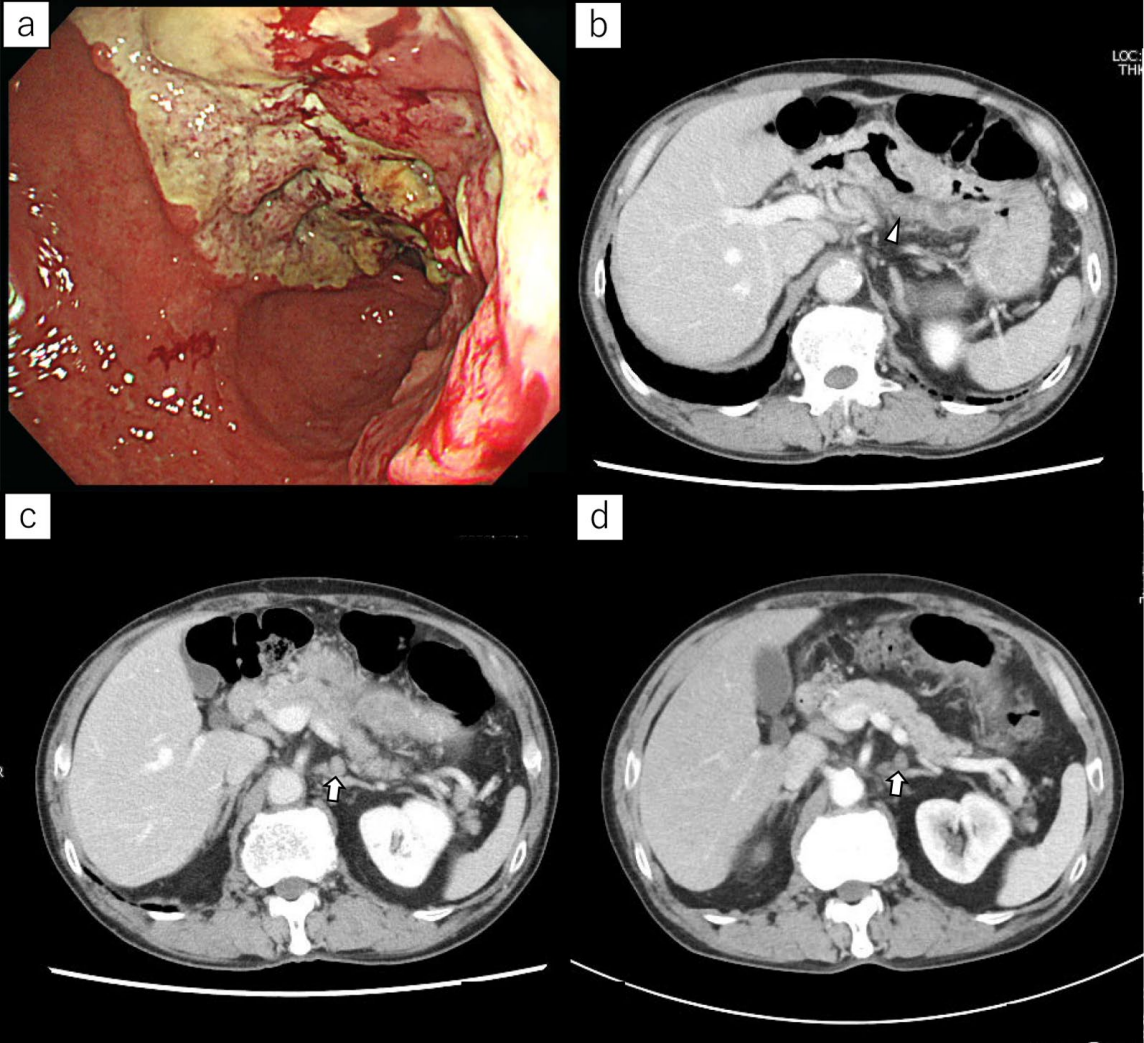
a Esophagogastroduodenoscopy revealed huge ulcerative lesion on the lesser curvature of stomach. b Abdominal CT revealed wall thickness of stomach (arrowhead) and enlarged lymph node along the left gastric artery and its branches. c Abdominal CT revealed enlarged para-aortic lymph node (arrow). d Para-aortic lymph node was slightly shrunk after chemotherapy (arrow)
